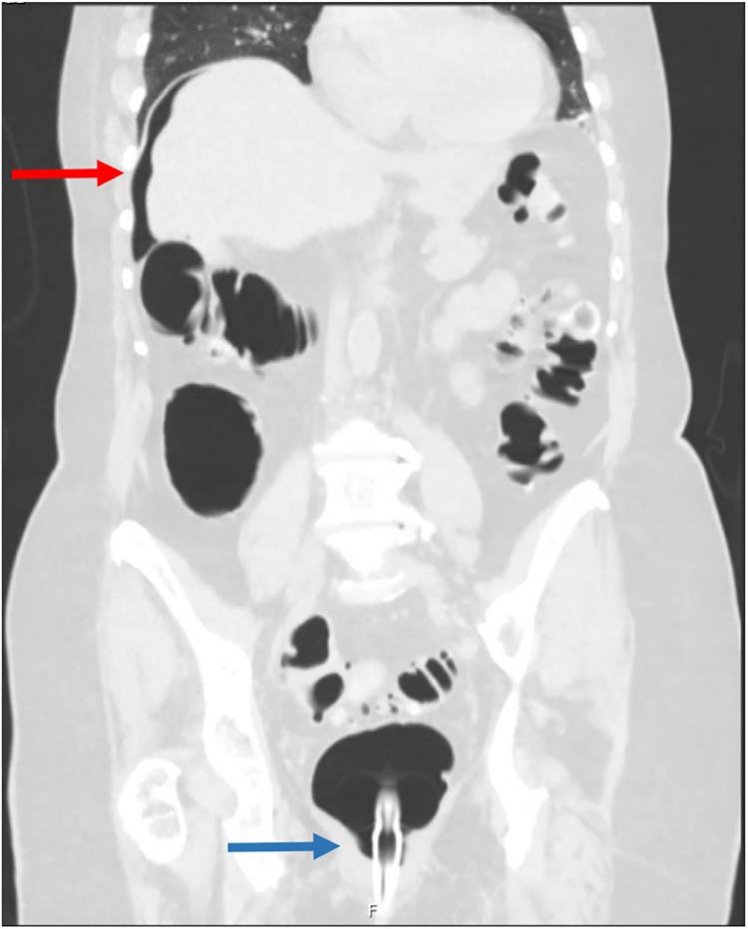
Coronal CT scan following gas insufflation; the red arrow points to pneumoperitoneum above the liver, and the blue arrow points to a rectal catheter.
